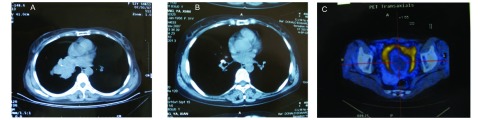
患者1的影像学特点。A：确诊时肺CT；B：化疗2周期后肺CT；C：PET-CT示腹腔代谢增高。 CT imaging of case one, 54-year-old woman with SCLC presented with a left ovarian metastases. A: lung imaging on CT when diag- nosed; B: lung imaging on CT after two circles of chemotherapy; C: pelvic cavity imaging on PET.
